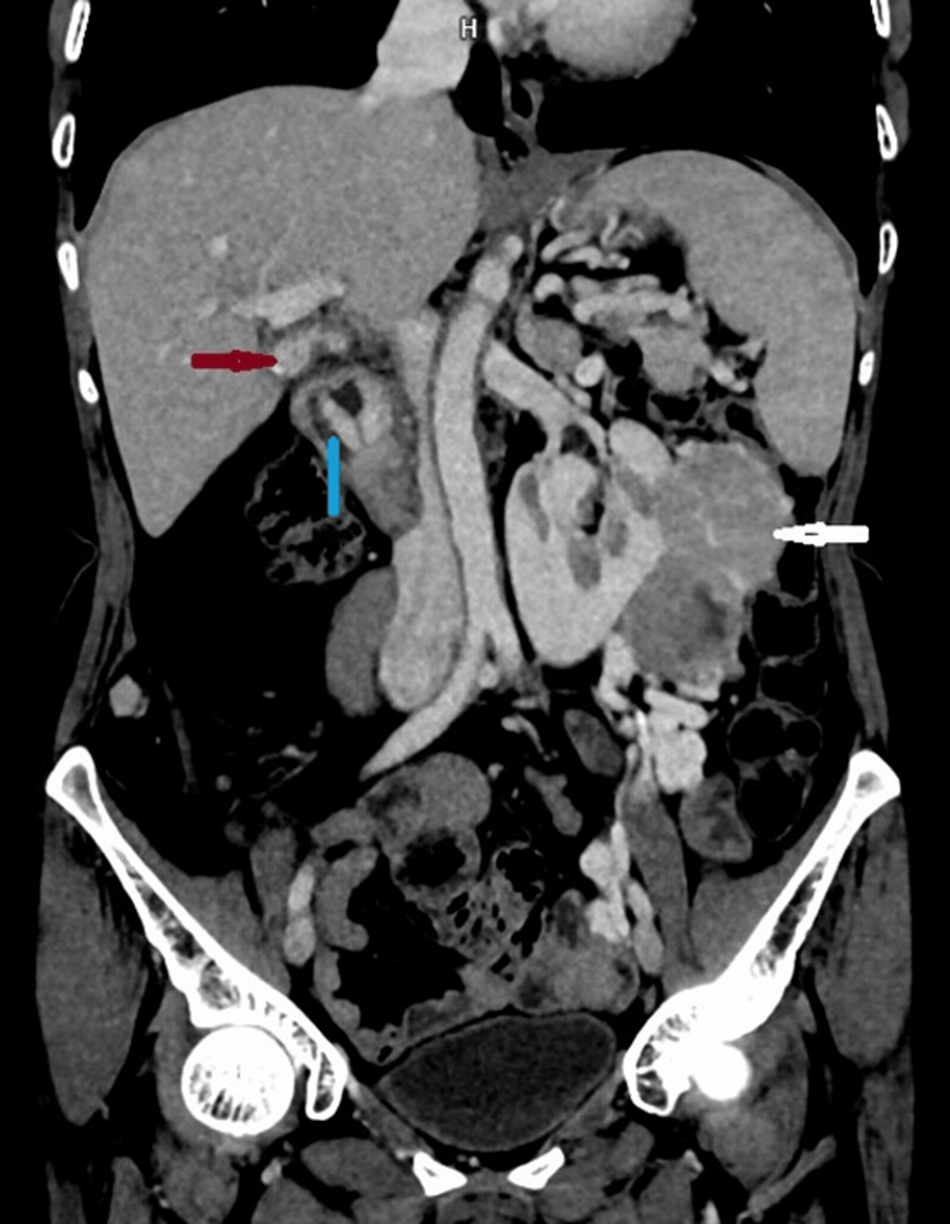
Coronal post-contrast computed tomography (CT) at the level of the left kidney Coronal contrast-enhanced CT shows an exophytic mass in the left moiety of the horseshoe kidney (white arrow). Also noted is an enhancing lesion in the gallbladder (red arrow) and duodenum (blue arrow).
